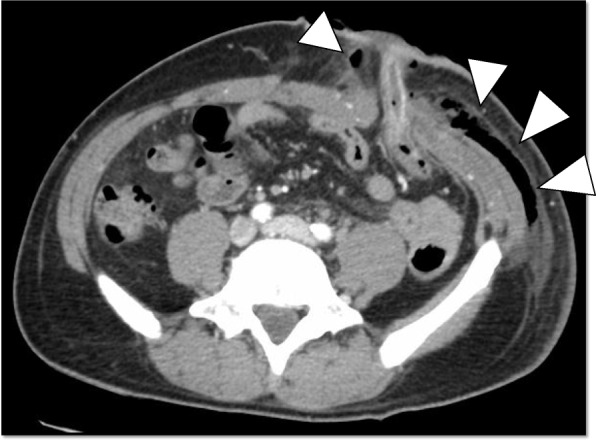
Initial computed tomography of the abdomen revealed an abscess formation with air around the colostomy (white arrows)
